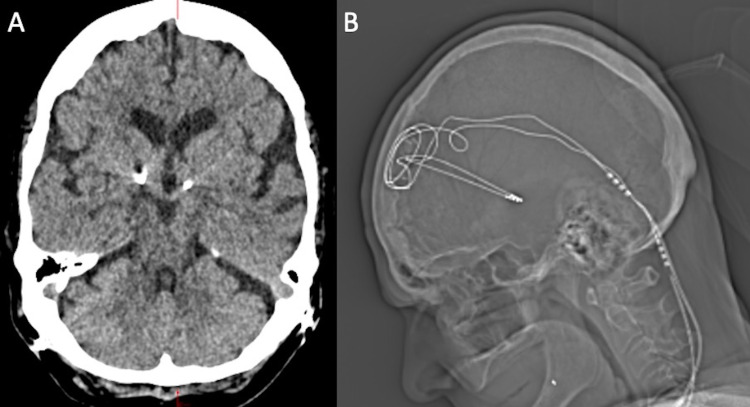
(A) Axial postoperative head CT demonstrating bilateral hypothalamic lead placement. (B) Lateral postoperative head x-ray demonstrating bilateral hypothalamic lead placement.
